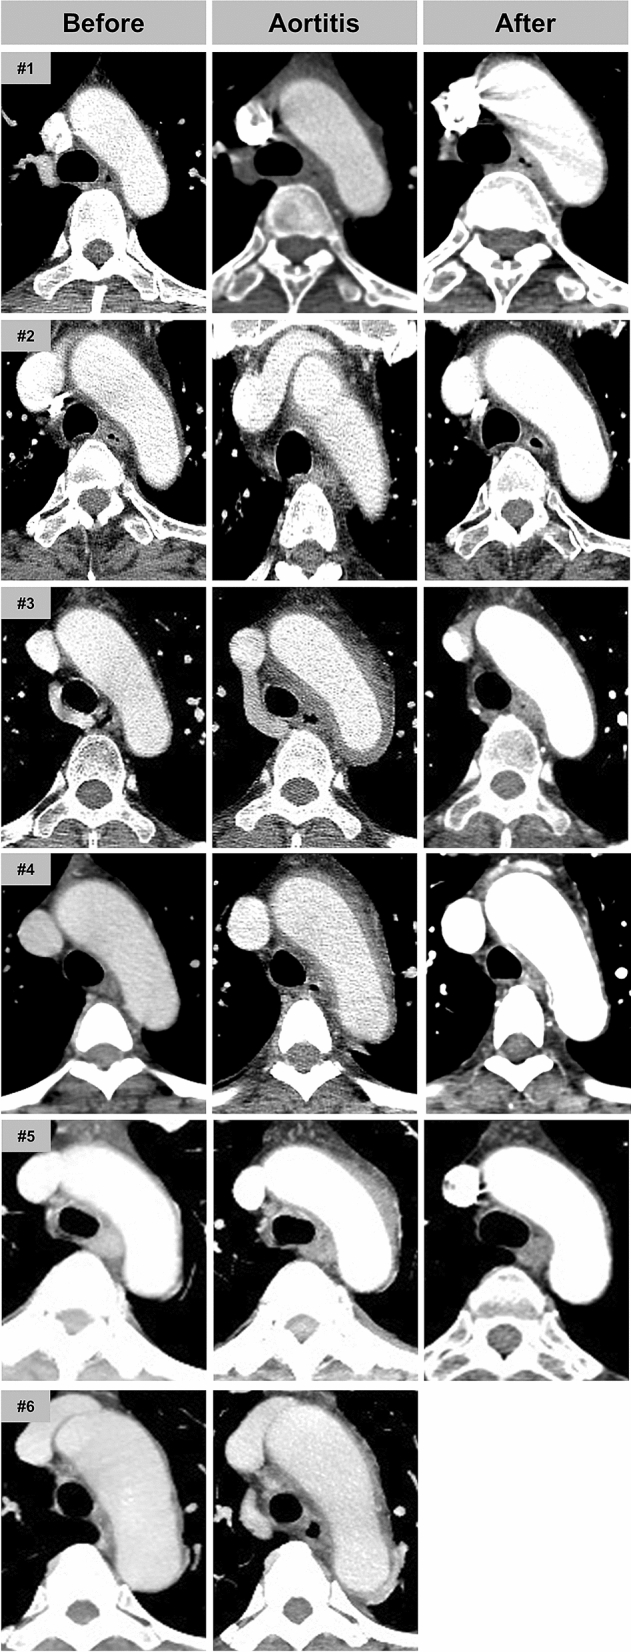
Serial CT images of aortic arch before, during and after PEGylated filgrastim-induced aortitis. Irregular wall thickening with periaortic infiltration is commonly observed in the aortic arch (Case #1–#6). After steroid treament, inflammatory changes of aortic wall were significantly improved.
